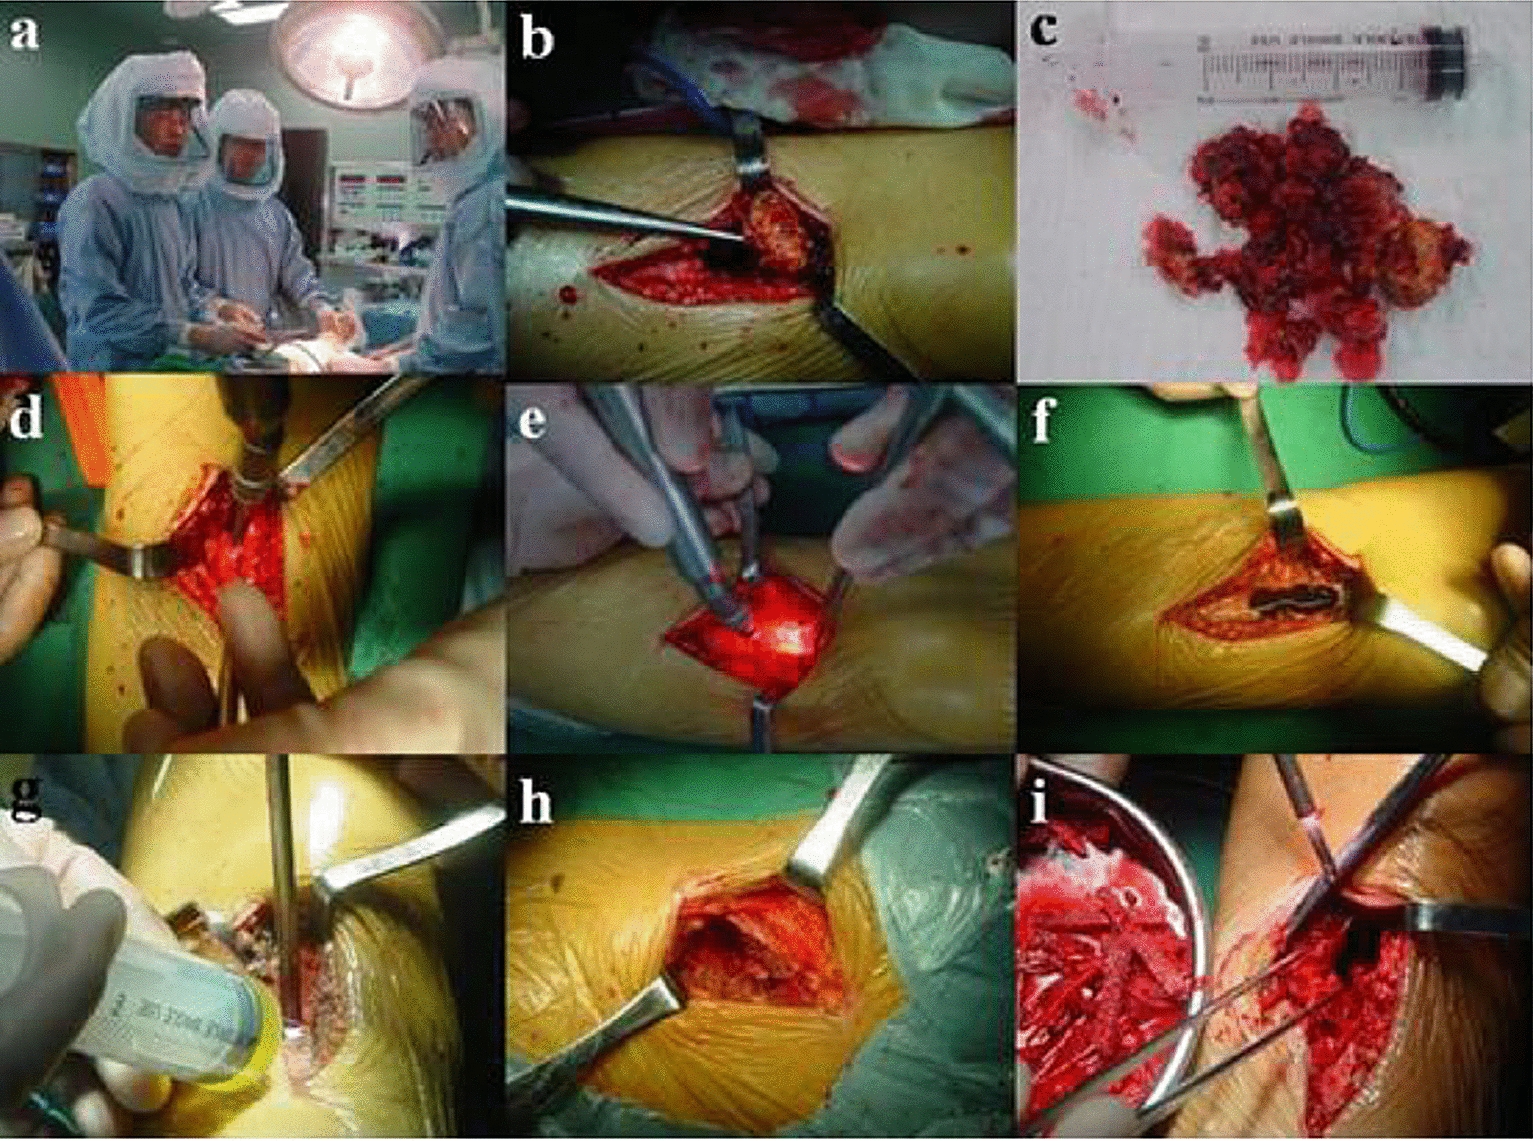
During the operation, the lesion was scraped and the bone cavity was polished with ultrasonic knife (a–f), the polished inner wall of the bone cavity was burned with anhydrous alcohol, and local methotrexate chemotherapy was performed (g), Autogenous iliac crest or allograft (h, i)
